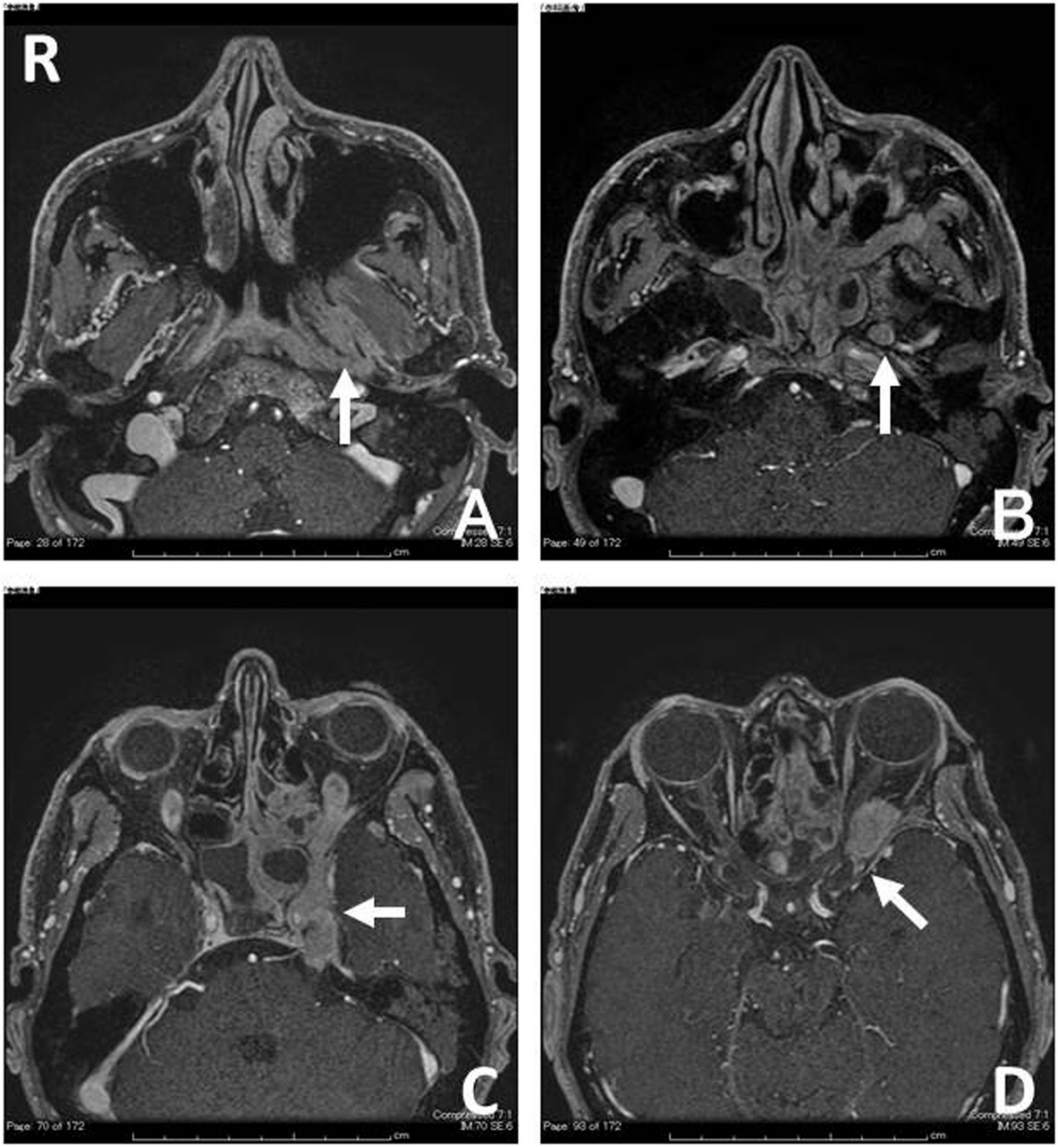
T1-weighted magnetic resonance imaging with gadolinium-diethylenetriaminepentaacetic acid showing a well-enhanced lesion in the fossa of Rosenmüller (A; arrowhead, R; right), the foramen ovale (B; arrowhead), and the left cavernous sinus (C; arrowhead) extending to orbital apex through the superior orbital fissure (D; arrowhead).
